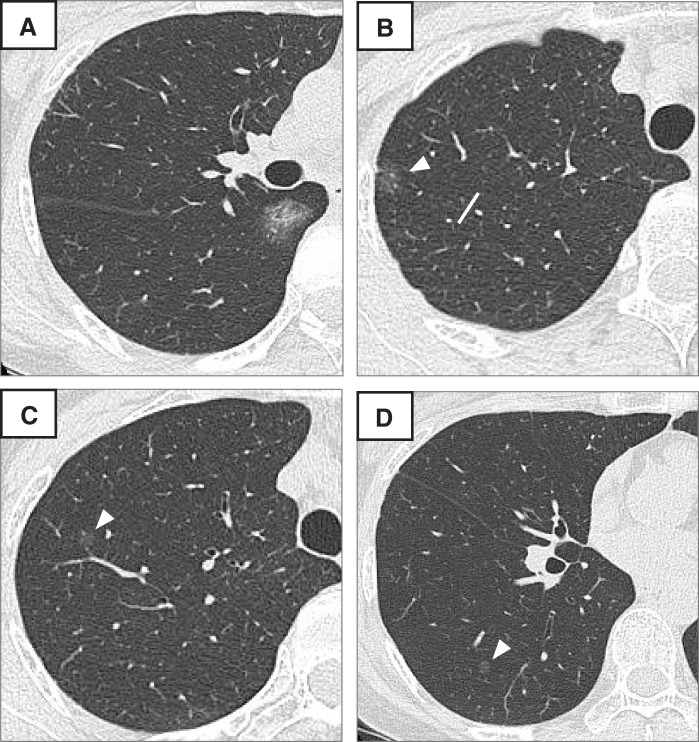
Preoperative CT images. (A) Axial CT showing a 22-mm part-solid ground-glass nodule located in the incomplete fissure between the right upper and lower lobes, with dense parenchymal fusion. (B) Axial image showing a 15-mm pure ground-glass nodule in the S2 segment (white arrowhead). (C) Axial image showing a 7-mm pure ground-glass nodule in the S2 segment (white arrowhead). (D) Axial image showing a 7-mm pure ground-glass nodule in the S6 segment (white arrowhead).
